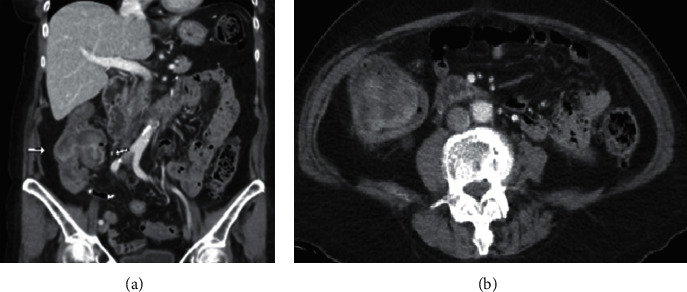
Right ascending mass in ascending colon CT. (a) (Arrows) colo-colonic intussusception with associated submucosal edema. (b) Right colonic mass measuring approximately 7 cm.
